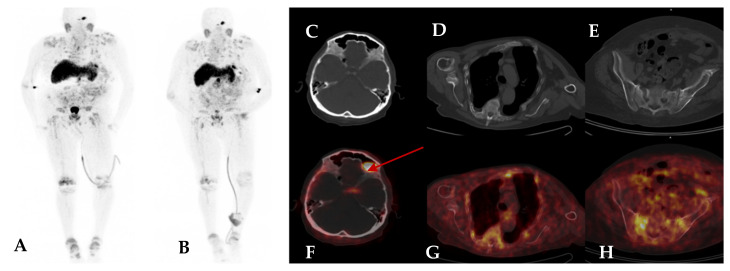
[^68^Ga]Ga-DOTATATE PET/CT images of a 66-year-old man with a 3.5-year history of osteoporosis and hypophosphataemia with multiple bone fractures (Case 6). The [^68^Ga]Ga-DOTATATE PET/CT (A) showed intense focal uptake in a meningioma in the left frontal sinus (SUVmax 21.1, red arrow), previously detected by MR, as well as in multiple bone fractures (with SUVmax up to 9.8), but no primary tumour was found ((A)—MIP image; (C–E)—CT images; (F–H)—transaxial PET/CT images). One year later, the follow-up [^68^Ga]Ga-DOTATATE PET/CT was nearly the same, except for some bone reparation process ((B)—MIP image).
